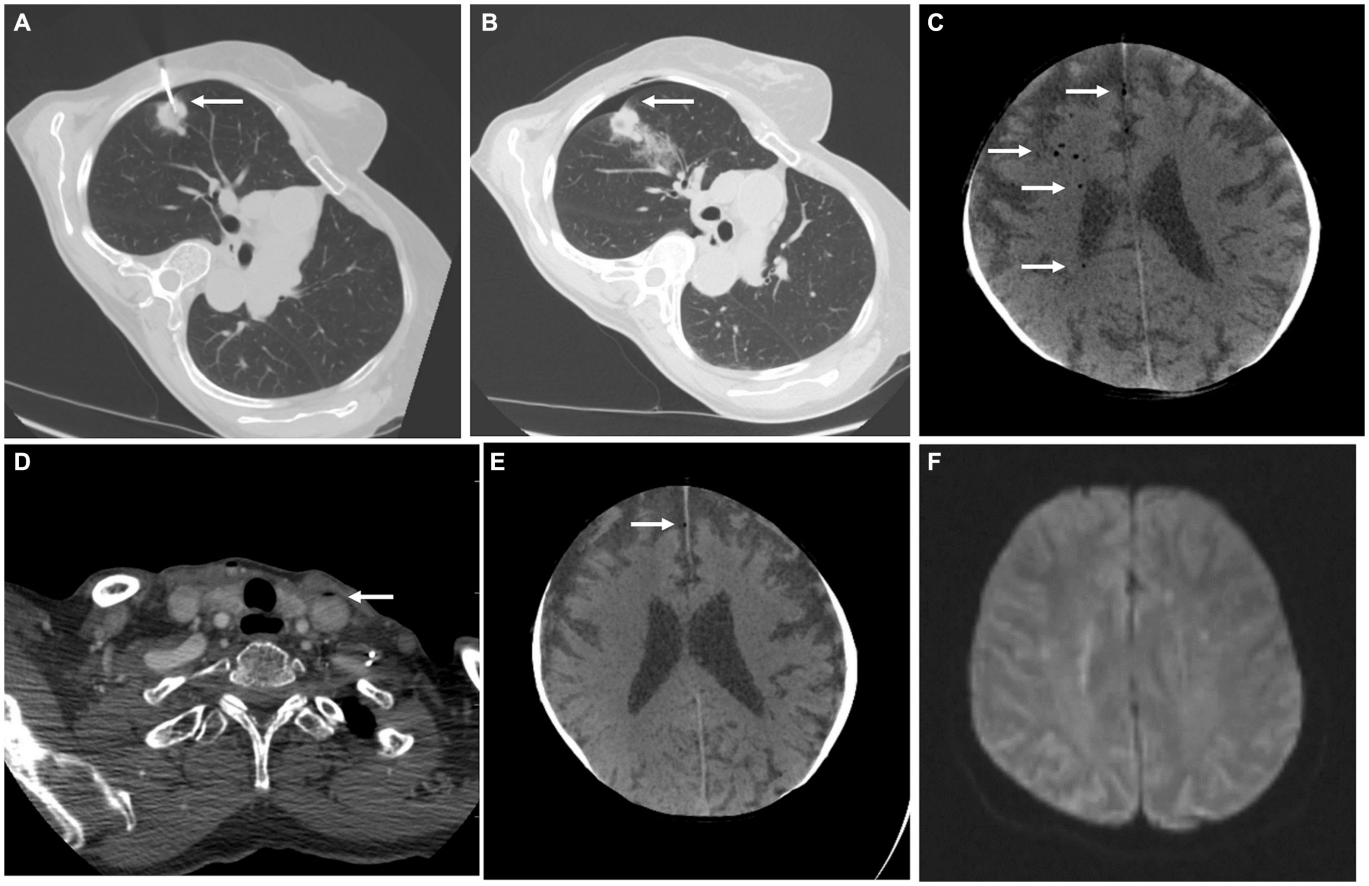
Imaging for cases 6 (A,B), 7 (C), and 11 (D–F). (A) Axial CTA scan of the brain with air bubbles in cavernous sinuses (white arrows). (B) CTA of the brain vasculature on the next day showing complete regression of the air emboli. (C) Axial CT scan of the brain in MinIP shows a very significant amount of air bubbles in the cerebral circulation of the right hemisphere (white arrows). (D) Axial brain CTA with occlusion of the M1 segment of the left middle cerebral artery (black arrow). (E) Axial native CT scan of the brain in MinIP shows a significant amount of air bubbles in the cerebral circulation in the left hemisphere (white arrows). (F) Native axial CT scan of the brain with developing extensive ischemia in the left middle cerebral artery territory, temporal, and occipital region (lower white arrow), and basal ganglia (upper white arrow). Air bubbles have disappeared.
